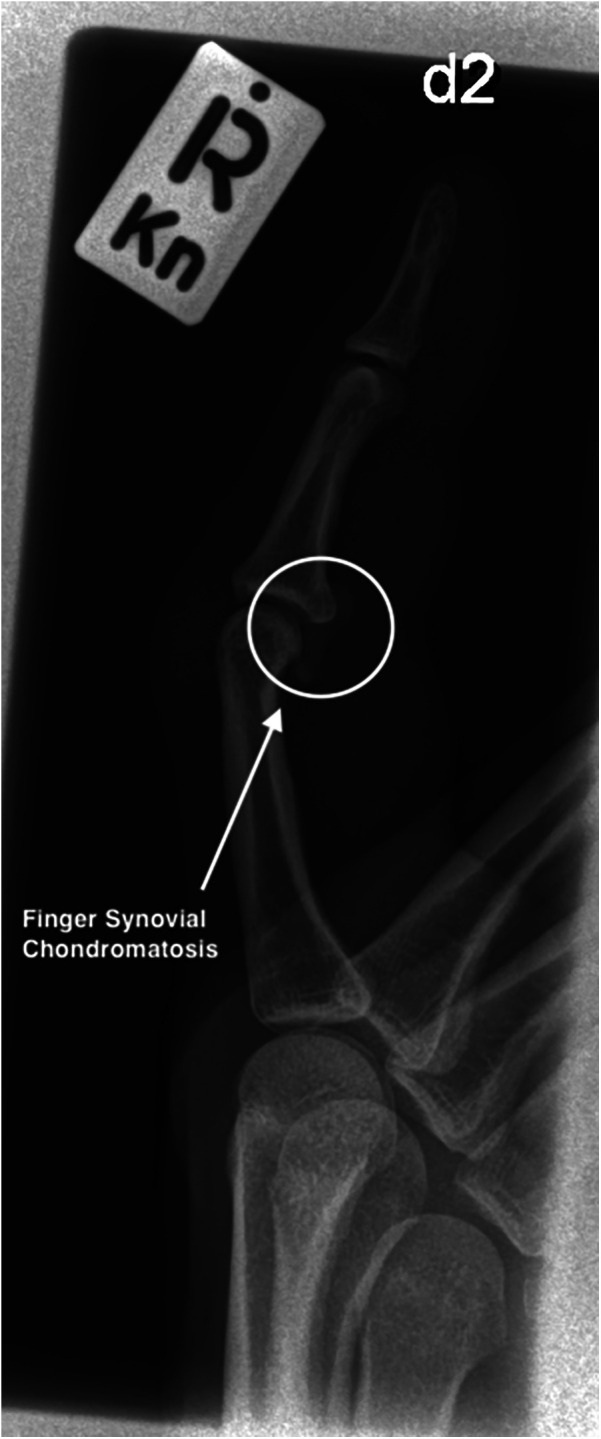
X-ray of the R D2 PIP head of 1st phalanx.
